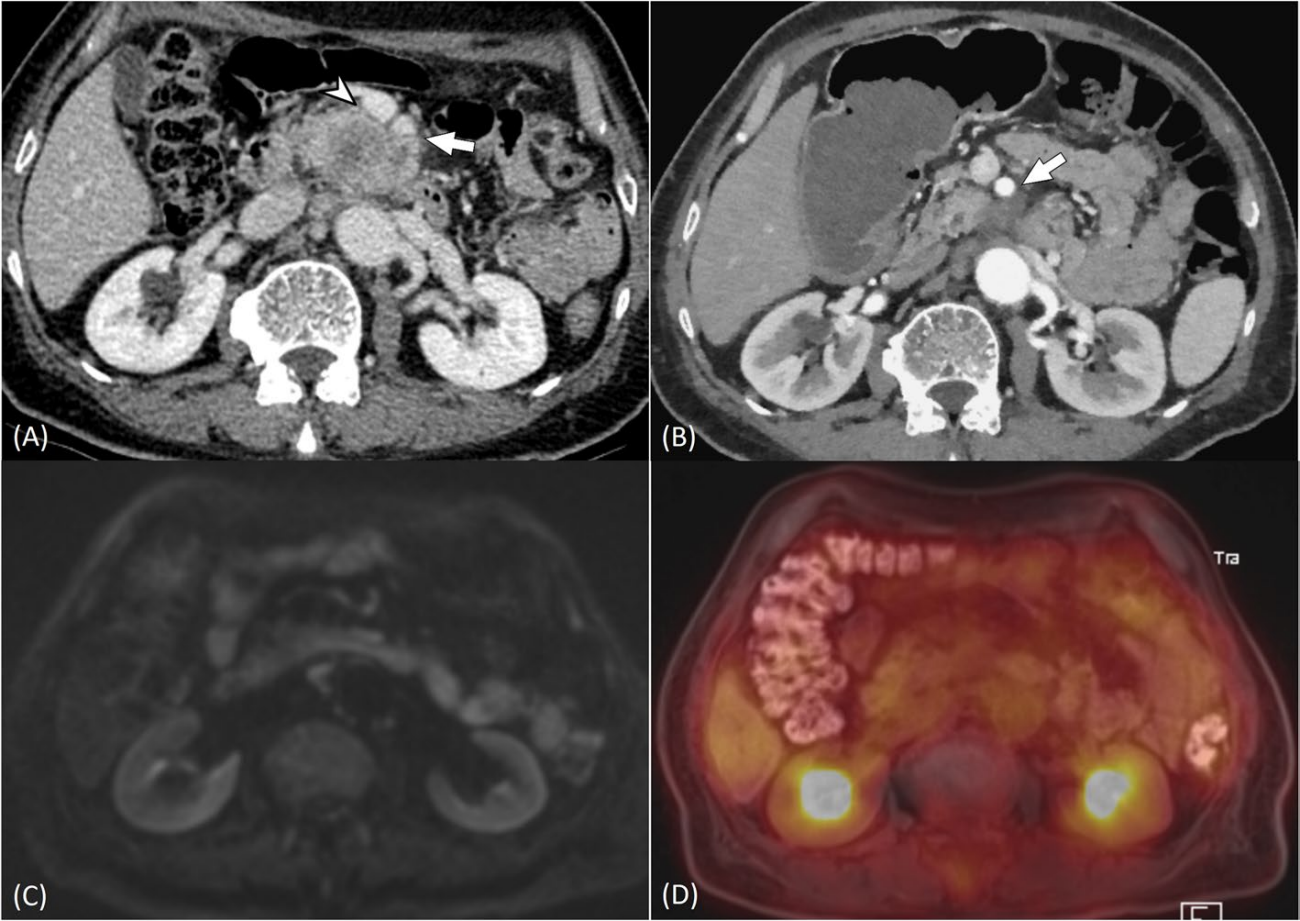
A 70-year-old man with pancreatic cancer. Axial portal venous phase image of baseline contrast-enhanced computed tomography (CECT) scan (A) shows a 5 cm-sized pancreatic cancer in the uncinate process, contacting > 180 degree with superior mesenteric artery (arrow) and causing contour irregularity of superior mesenteric vein (arrowhead). On arterial phase image of post-neoadjuvant therapy (NAT) CECT scan (B), pancreatic cancer showed decrease in size to 3 cm and ≤ 180 degree contact with superior mesenteric artery (arrow), and contour irregularity of superior mesenteric vein was resolved. CECT resectability score was 3 (indeterminate resectability) according to the three reviewers in consensus. Since there was no diffusion restriction on diffusion-weighted imaging (DWI) (C) and no fluorodexyglucose (FDG) avidity on. ^18^F-FDG-positron emission tomography (PET) (D) at tumor-vessel contact of post-NAT PET/MRI, and carbohydrate antigen level (CA) 19–9 was 3284 U/mL at initial diagnosis which reduced to 4 U/mL after NAT, the reviewers modified the resectability score to 4 (probably resectable) on both CT plus PET/MRI set and CT plus PET plus CA 19–9. The patient underwent Whipple’s surgery, and pathologic analysis showed no residual tumor with ypT0N0
